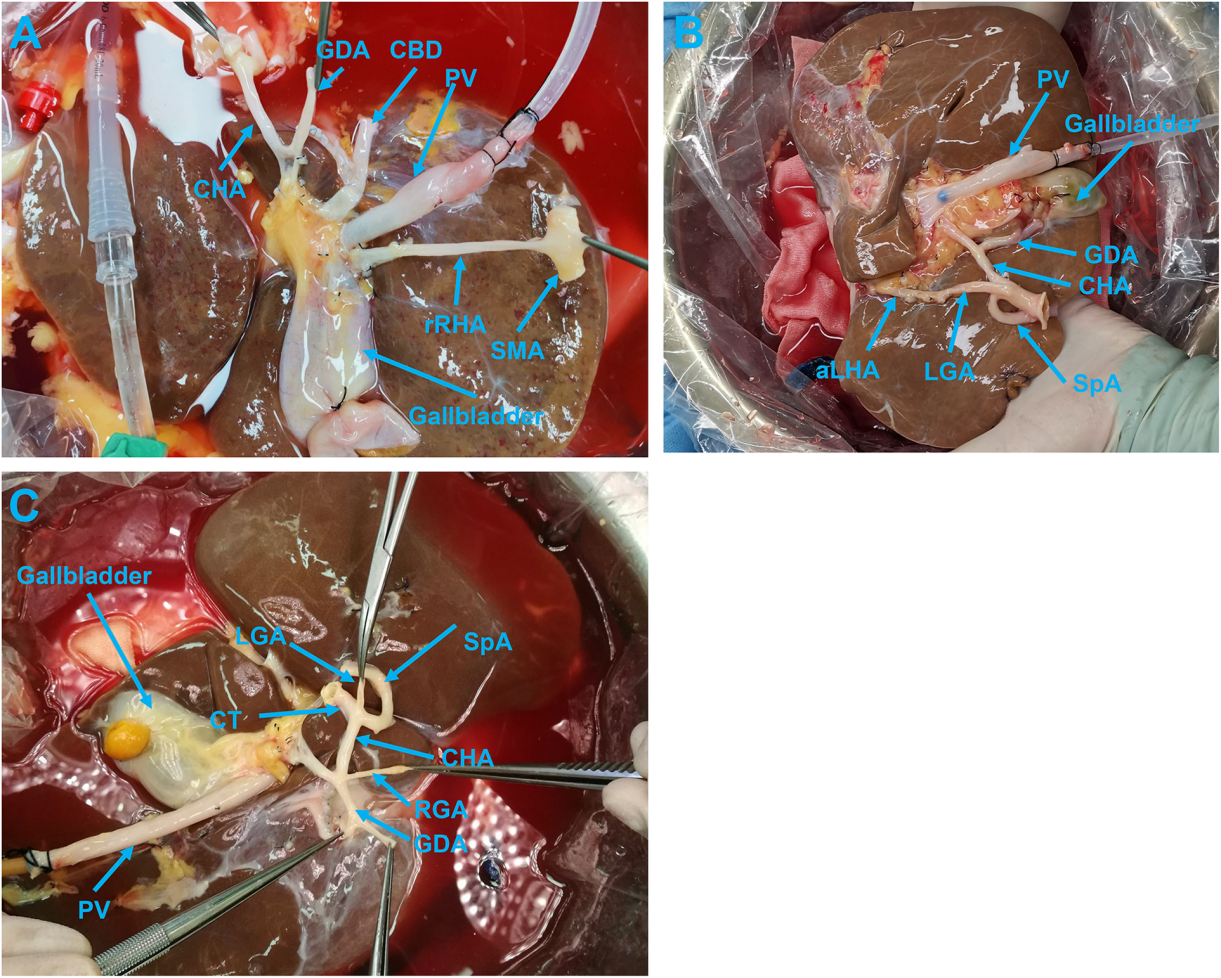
(A) A donor liver with high-quality preparation of aberrant hepatic artery. (B) Donor liver with an aberrant hepatic artery showing high-quality back-table preparation of arteries. (C) Donor liver without an aberrant hepatic artery showing high-quality back-table preparations of arteries. aLHA, accessory left hepatic artery; CBD, common bile duct; CHA, common hepatic artery; CT, celiac trunk; GDA, gastroduodenal artery; LGA, left gastric artery; PV, portal vein; RGA, right gastric artery; rRHA, replacement right hepatic artery; SMA, superior mesenteric artery; SpA, splenic artery.
